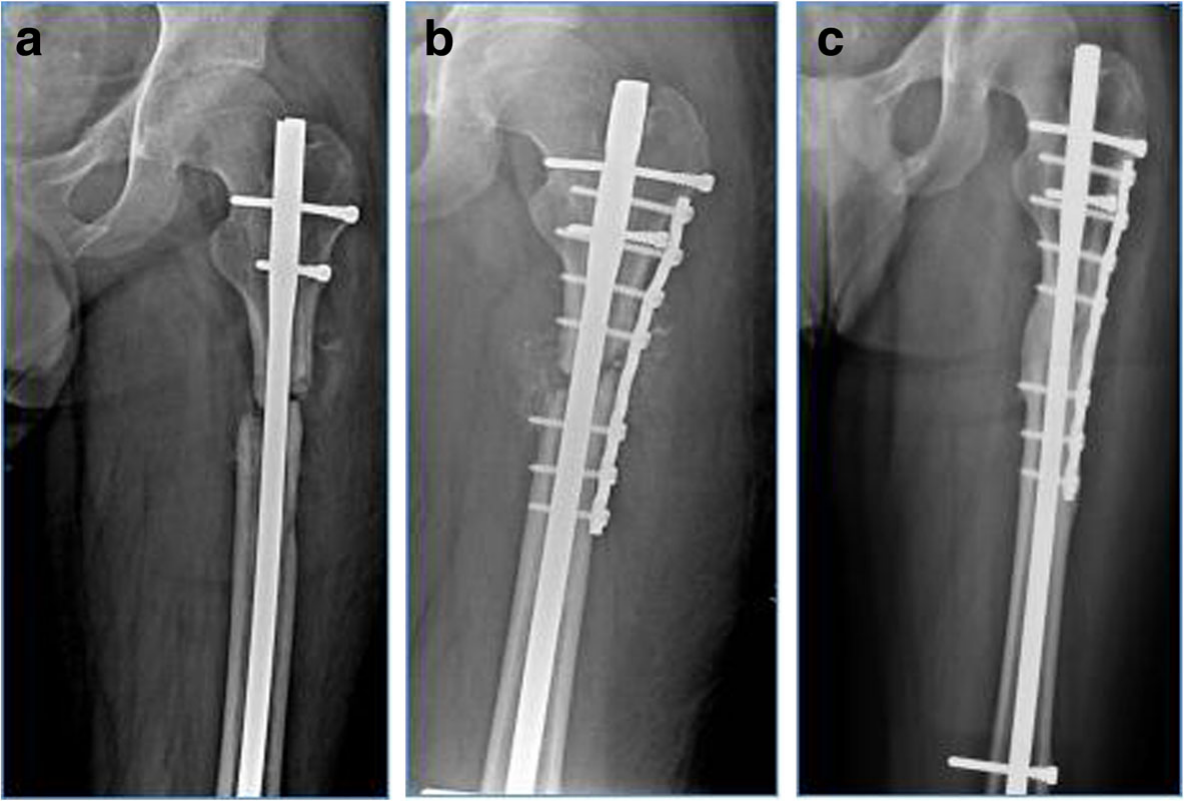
A male patient, aged 39, suffered from femoral shaft supraisthmal fracture following a traffic accident. a Anteroposterior X-ray showed femoral shaft nonunion 9 months after treated with antegrade IMN. b Instant radiography after primary revision by ACP associated with ABG procedure. c Complete bone healing was observed 4 months after revision surgery.ABG, autogenous bone grafting; ACP, augmentative compression plating; IMN, intramedullary nailing
